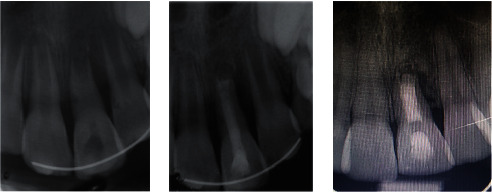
(a) Periapical radiograph showing the initiation of external root resorption on the left central maxillary incisor after 6 weeks. (b) Placement of an MTA plug and root canal obturation with gutta-percha after the resorption process ceased 9 months later. (c) The same tooth at 10-year follow-up.
